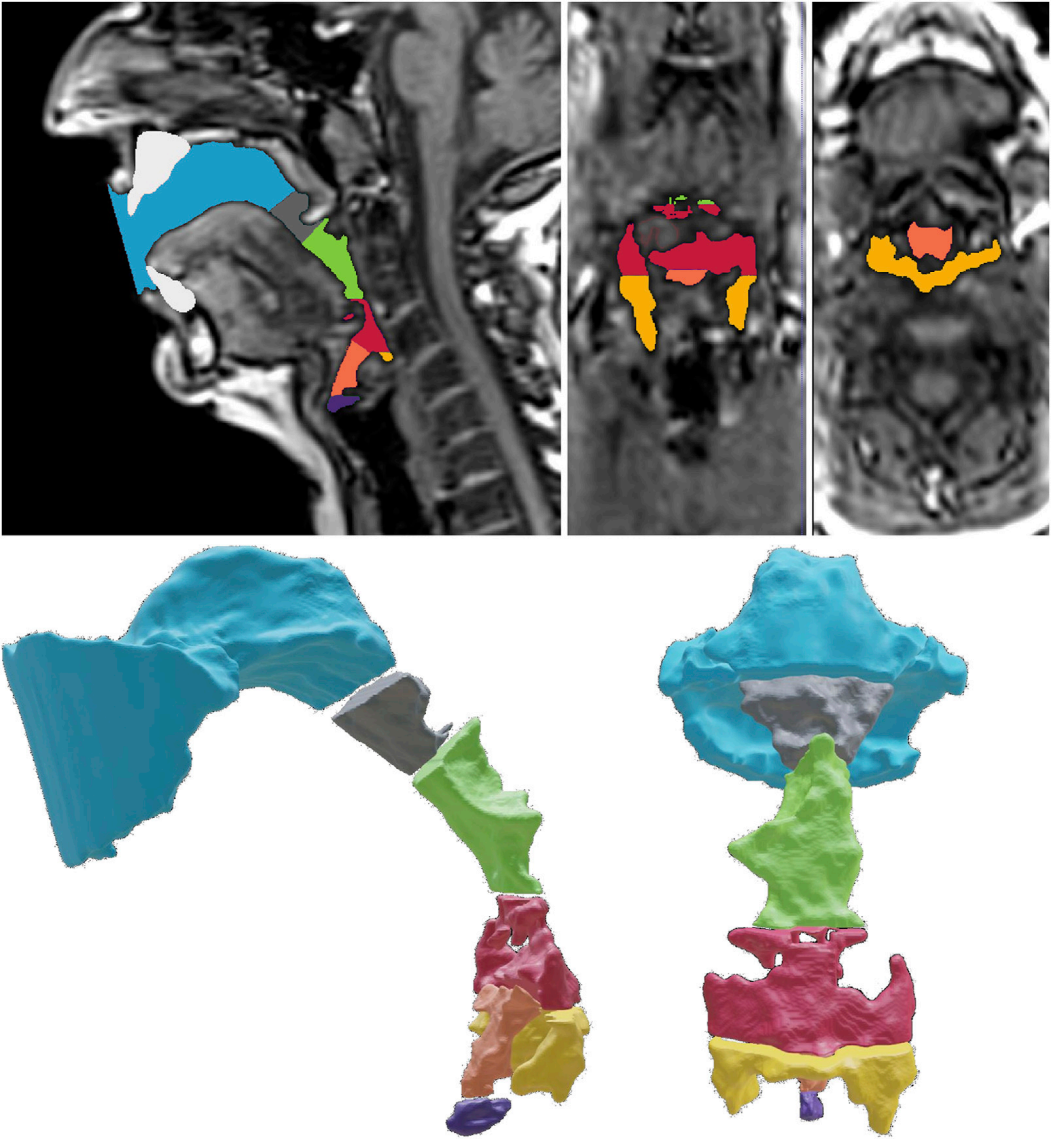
Definition of seven sub-volumes needed for the volumetric analysis of the sub-volumes (exemplarily shown for Belting /aː/ (A♭3). The upper row shows the mid-sagittal, coronal, and the transversal plane of the MRI data and the segmented cavities. The lower row shows the exploded view of the sub-volumes in the sagittal and coronal view. The blue color is used to highlight the oropharynx front (OPf), and the oropharynx rear (OPR) is shown in gray. The upper hypopharynx (HPu) is highlighted in green, whereas the lower hypopharynx (HPl) is highlighted in red. Finally, the epilarynx tube (ET), *Sinus piriformis* (SP), and ventricular volume (VV) are shown in orange, yellow, and purple, respectively.
